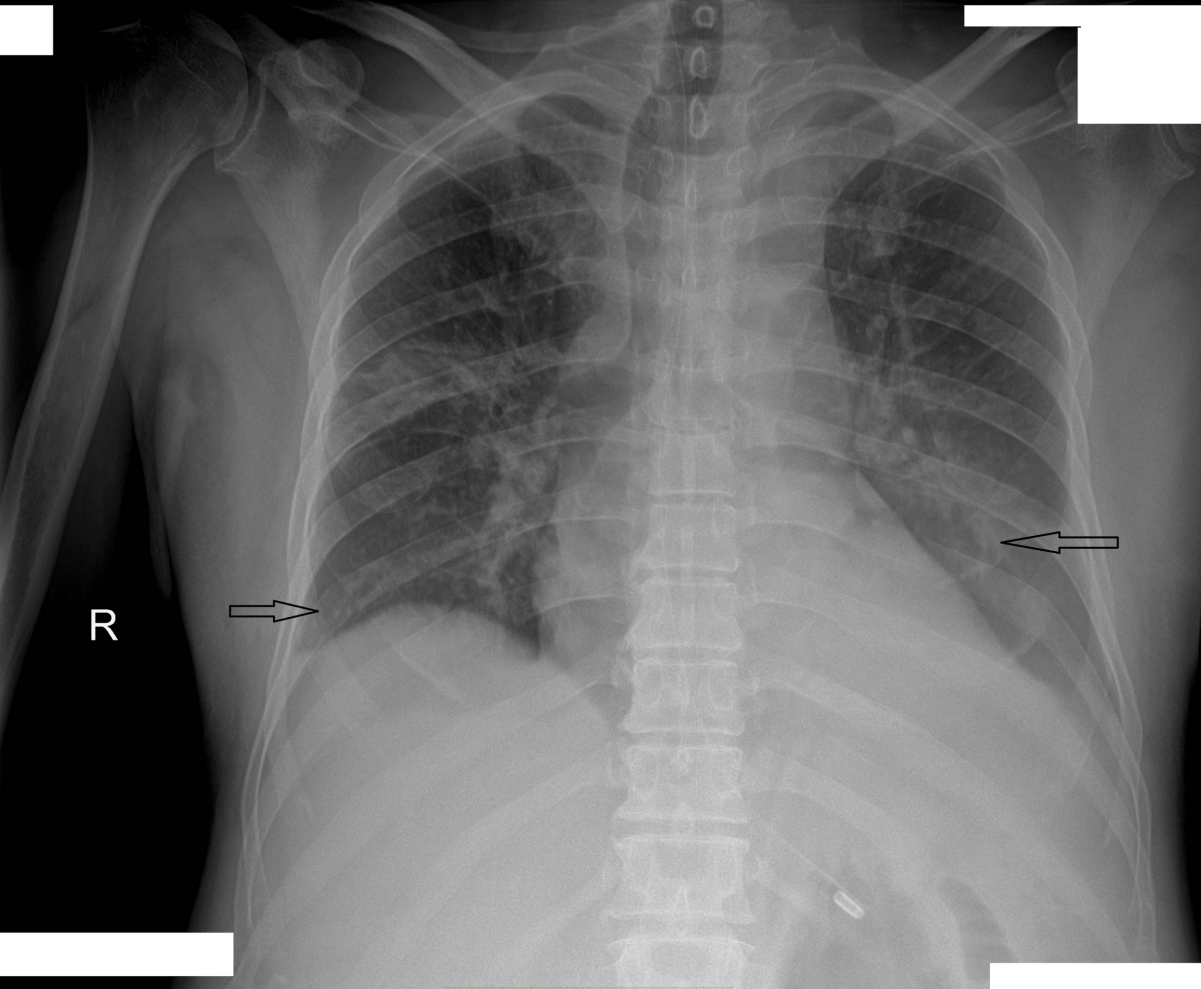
Chest X-ray revealing bilateral lower lobe consolidation
